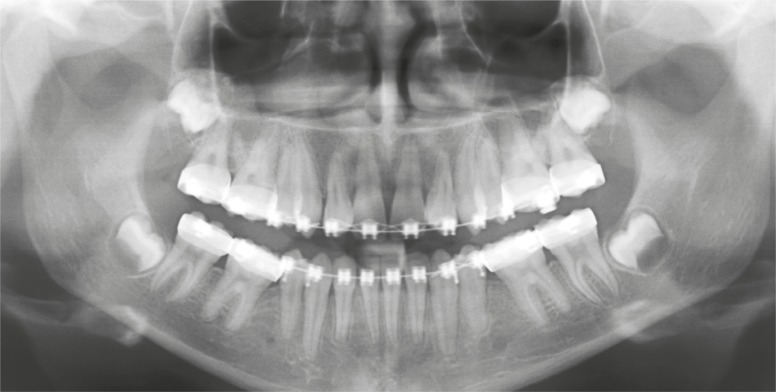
Panoramic radiograph taken during treatment.
